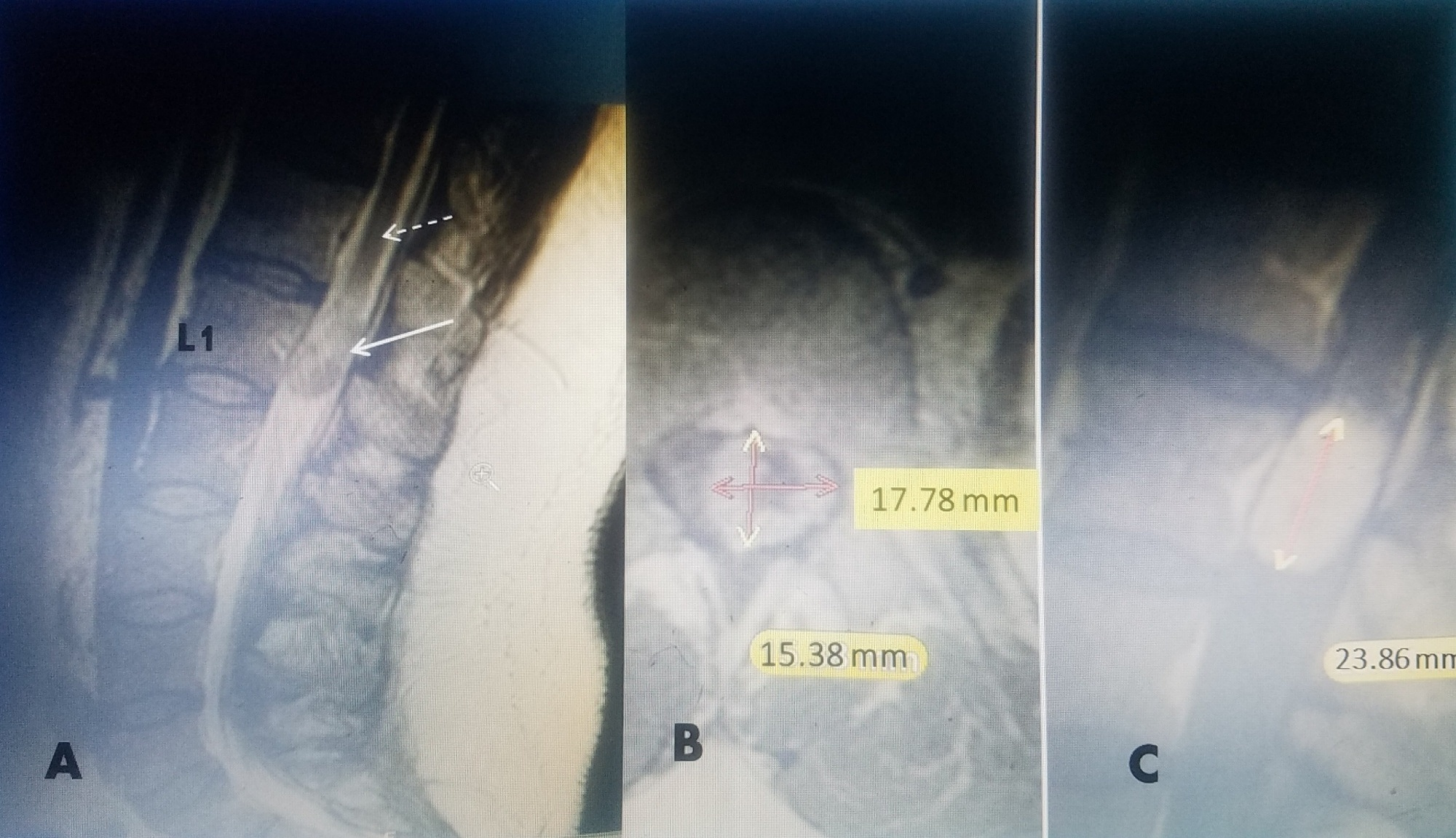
A 45-year-old male with incidental finding of conus medullaris mass. A: T2 sagittal magnetic resonance imaging (MRI) without gadolinium (Ga^+^) showing mixed density expansile mass in conus medullaris. B: Post-Ga^+ ^T1 MRI axial image showing hypodense center with increased peripheral uptake 17.78 millimeters (mm) coronal and 15.38 mm anterior-posterior dimension. C: Post-Ga^+ ^Sagittal T1 with contrast showing isodense 23.86 mm sagittal dimension. Total volume is 6.54 cubic centimeters (cm^3^).
